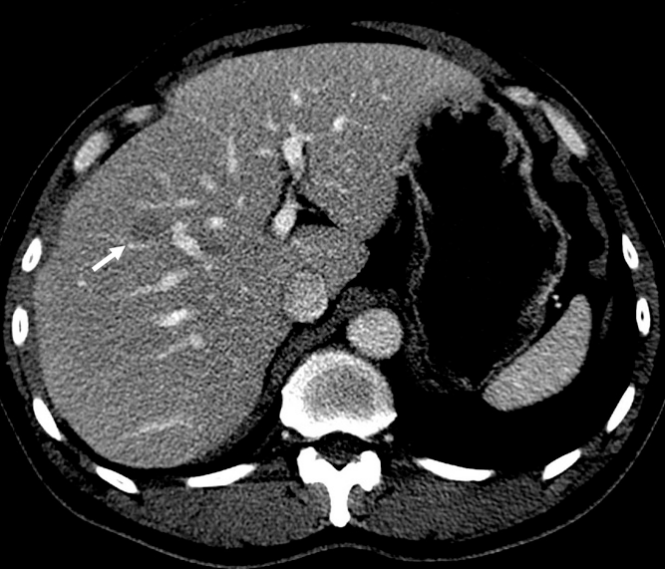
The CT image obtained 6 months later shows that the nodule migrates slowly upward and anteriorly in the liver (arrows).
